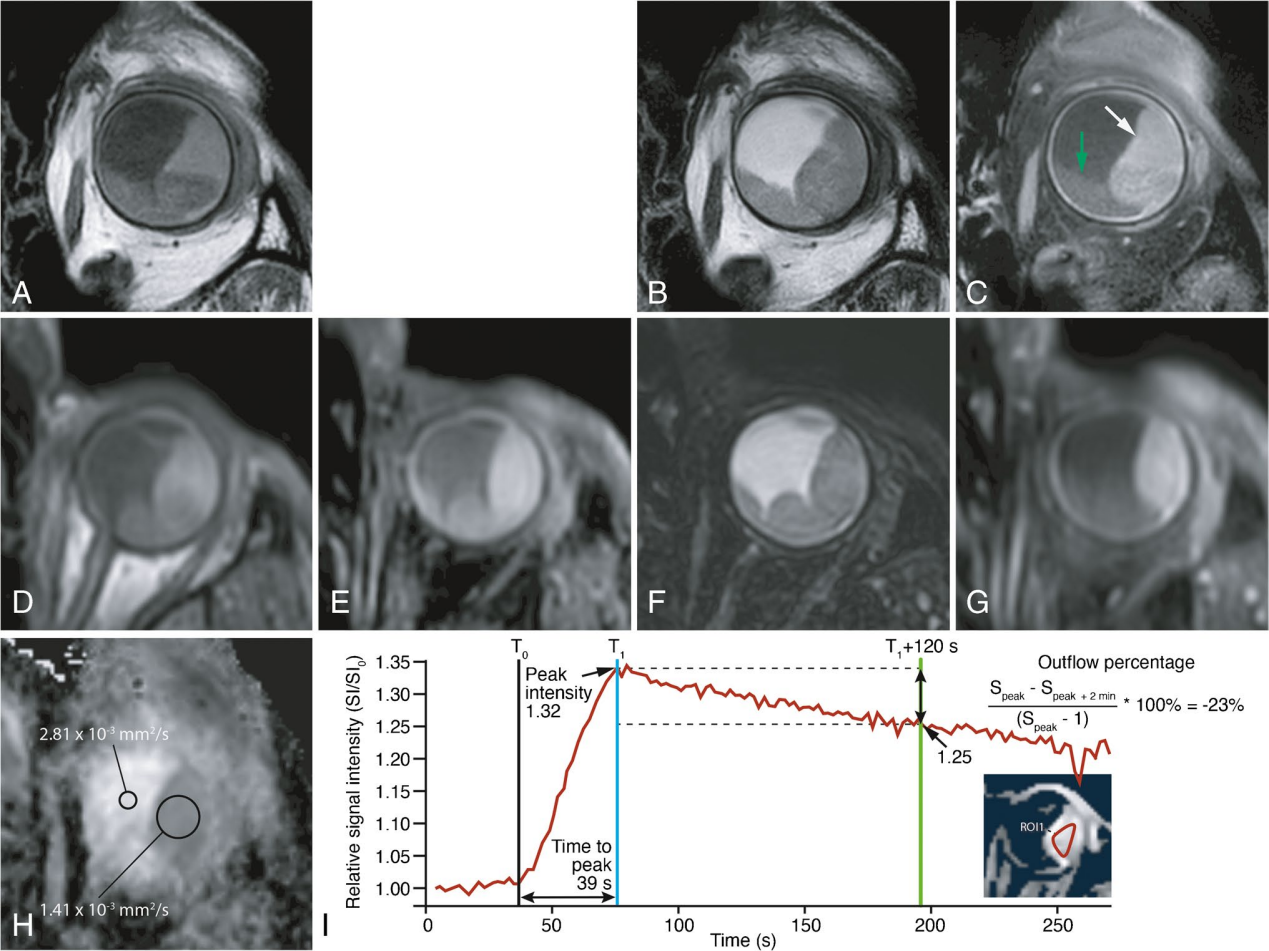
MRI ocular protocol. Uveal melanoma of the left eye (white arrow) with associated retinal detachment (green arrow). ADC of 1.4 × 10^−3^ mm^2^/s. Wash-out time-intensity curve at DCE. A MS T1. B MS T2. C MS contrast-enhanced T1 with fat signal suppression. D 3D TSE T1. E 3D TSE T1 with fat signal suppression. F 3D TSE T2 with fat signal suppression. G 3D TSE contrast-enhanced T1 with fat signal suppression. H ADC. I Quantitative evaluation of the DCE. Black line—arrival time (T0) = timepoint at which the lesion starts to enhance, determined manually. Blue line—corresponds approximately to the peak time (T1) = first timepoint when the lesion reached 95% of its maximum intensity, determined automatically and corresponding to the timepoint at which peak intensity (PI) is calculated. Time to peak (TTP) (s) = T1-T0. Green line—outflow percentage at 2 min (OP2,%) = percentage of signal intensity loss at 2 min compared to the intensity at the peak time. Notice the wash-out time-intensity curve. SI, signal intensity at every timepoint. SI_0_, signal intensity at timepoint zero
